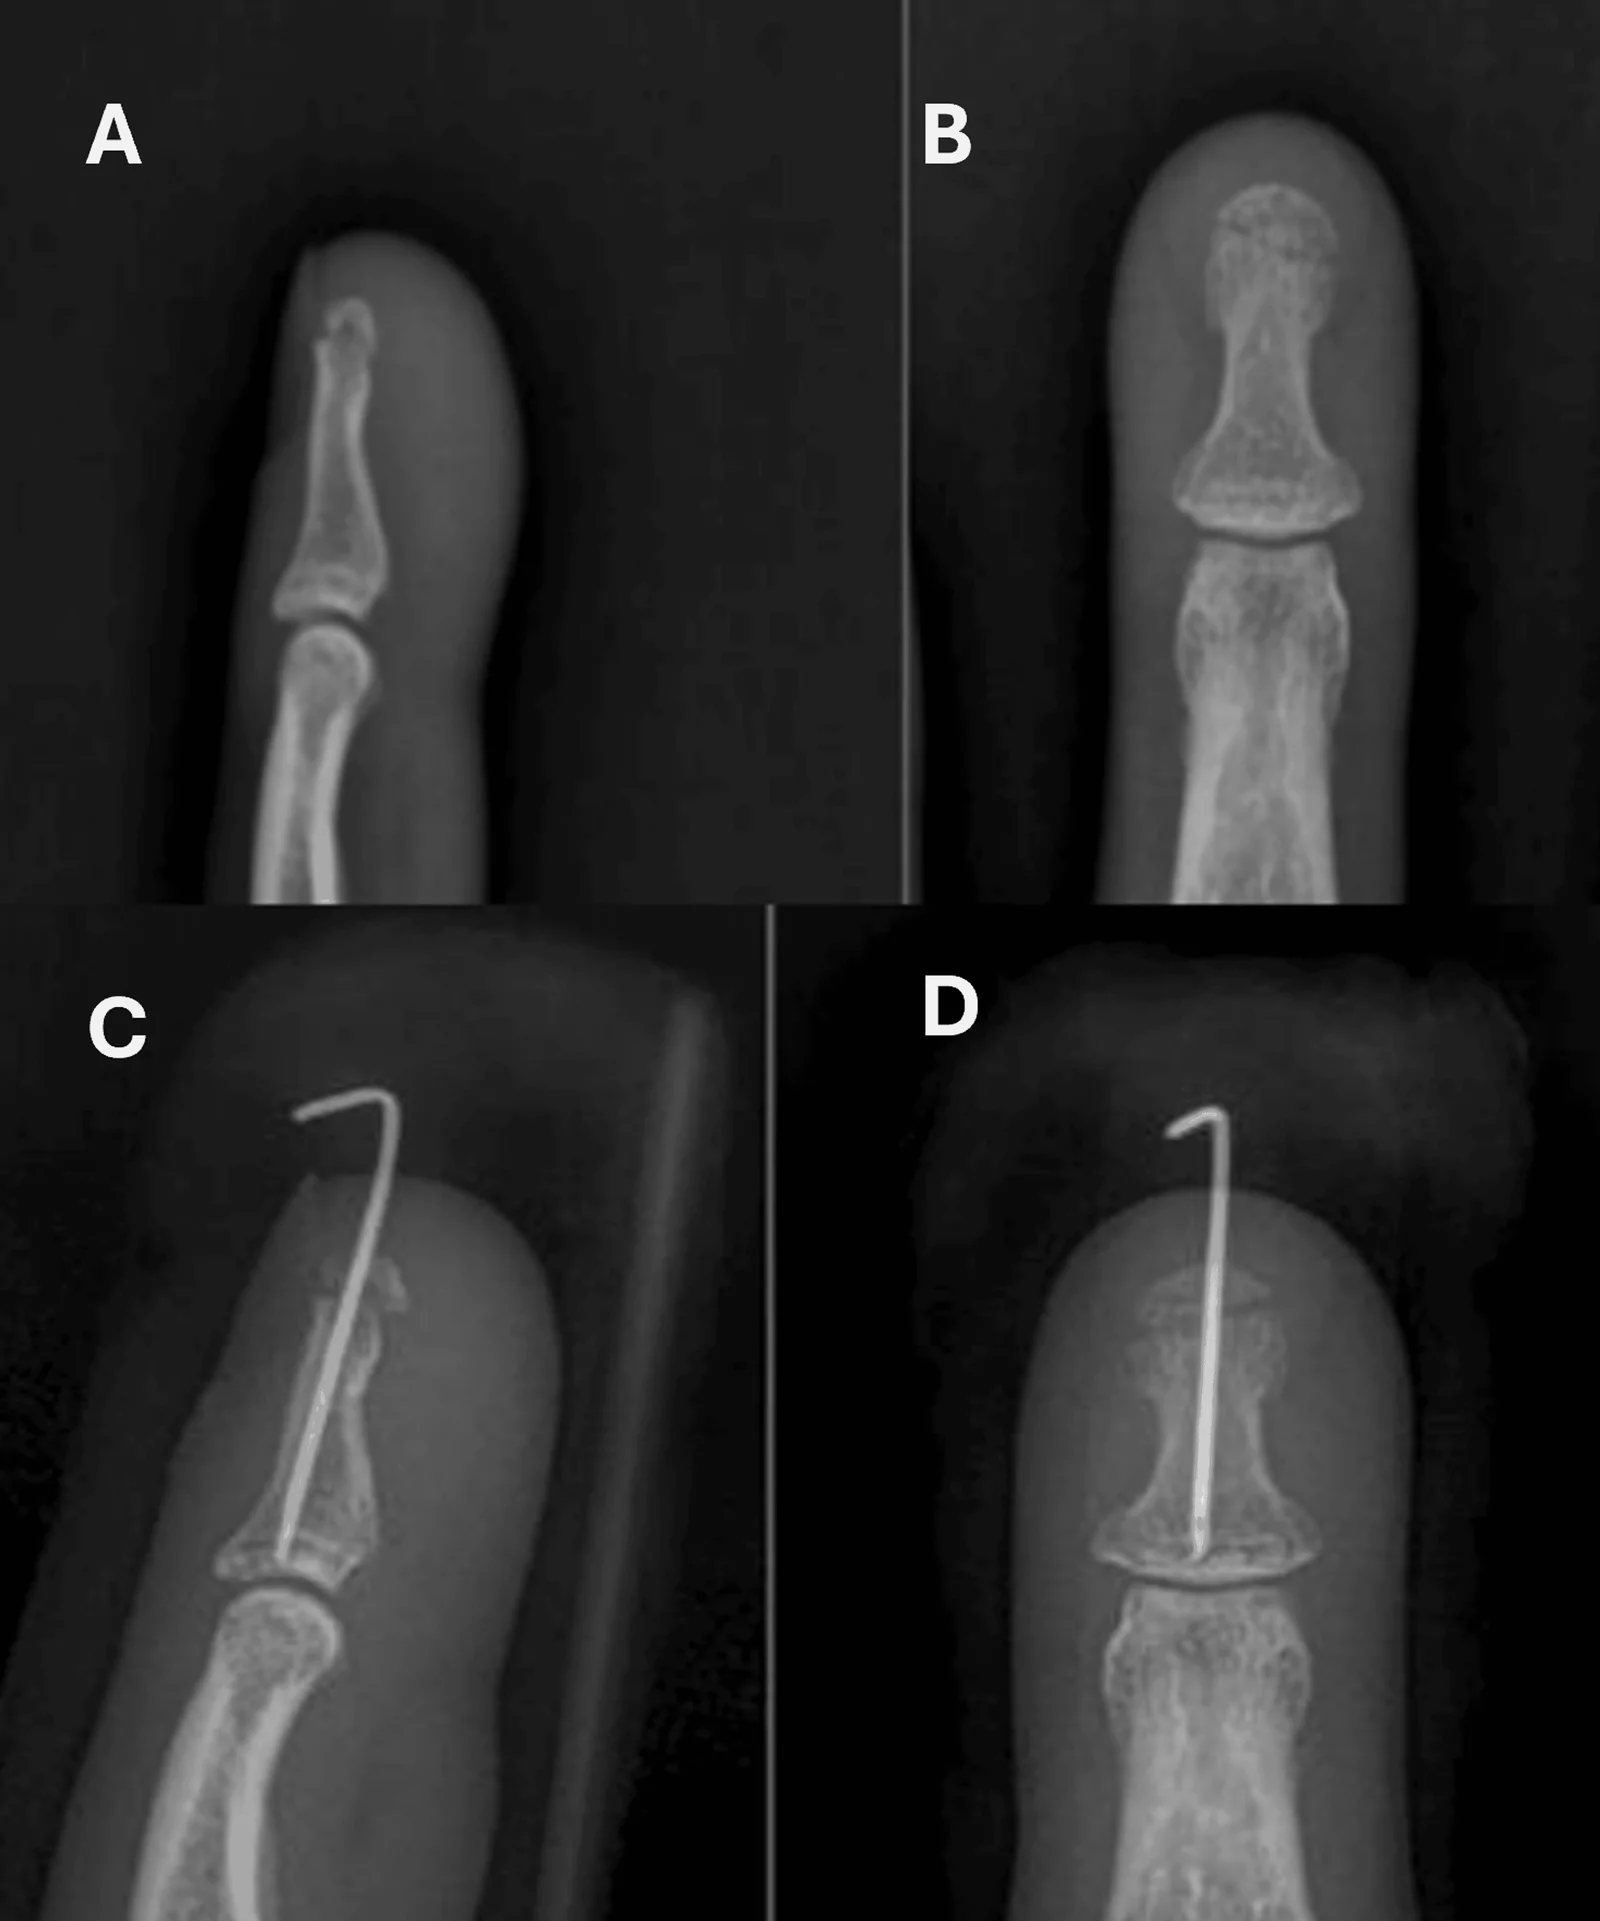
Plain radiographs of the dominant left middle finger. (A) Pre-intervention lateral view. (B) Pre-intervention anteroposterior (AP) view showing the open distal phalanx fracture. (C) Immediate post-procedure lateral view. (D) Immediate post-procedure AP view demonstrating anatomical reduction and stabilization achieved with a single 18-gauge hypodermic needle.
